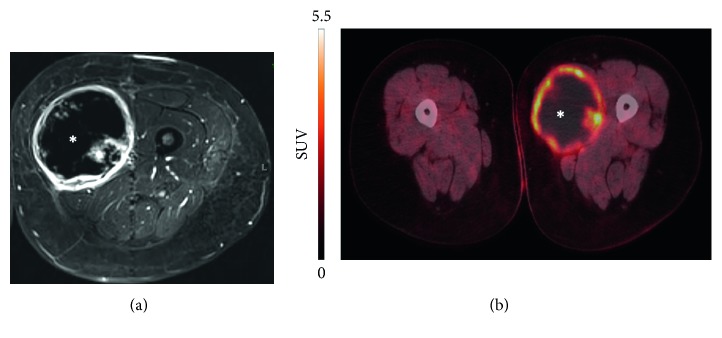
Example of a malignant peripheral nerve sheath tumor in the adductor musculature of the left thigh visualized using (a) magnetic resonance imaging (MRI) and (b) positron emission tomography (PET) studies. The hypointense areas marked with the asterisk represent necrosis within the tumor.
